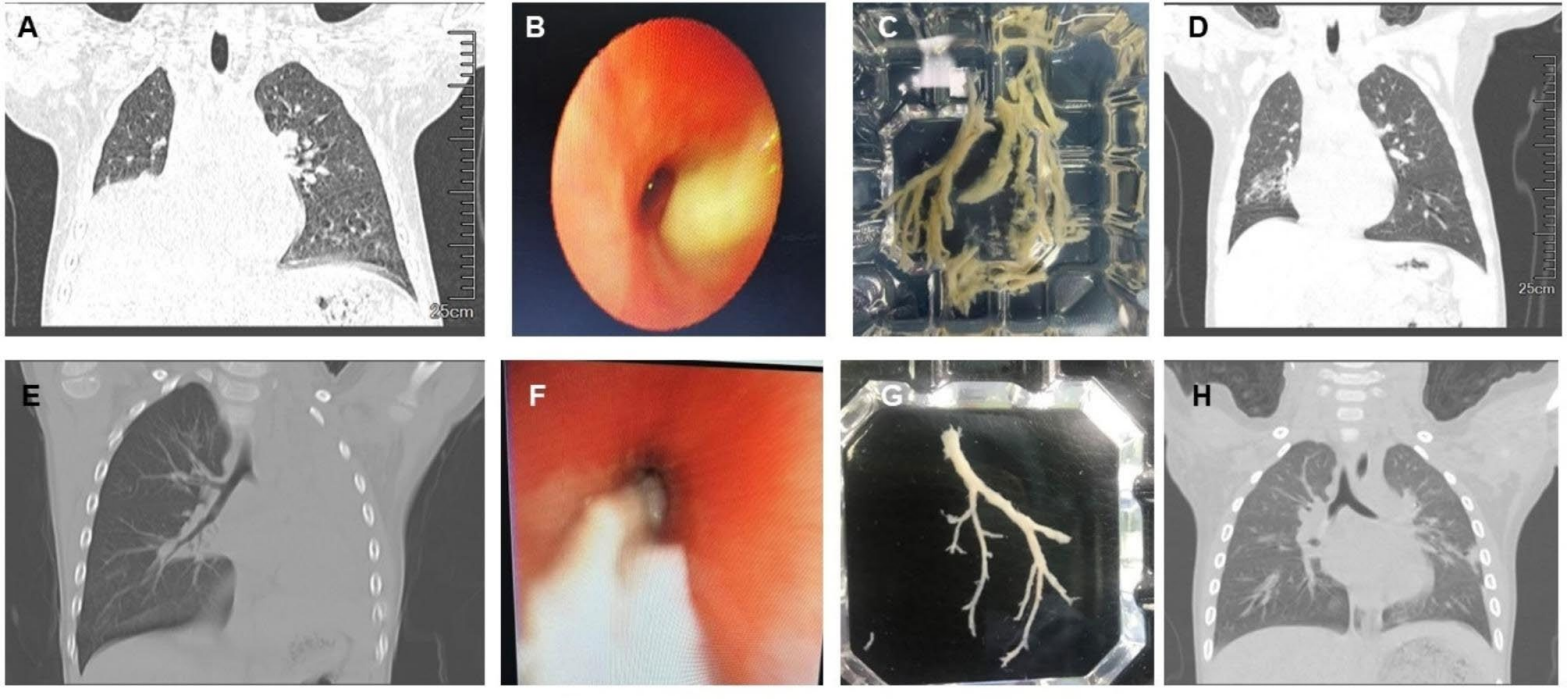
The relevant radiological and bronchoscopy images. Images A-D represent a 5-year-4-month-old girl diagnosed with MP Infection. A: Chest CT on admission showed large consolidated inflammation in the right lung, accompanied by atelectasis of the right lower lobe; B: Bronchoscopy revealed mucus plug obstructing the right lower lobe bronchus; C: The mucus plug was successfully removed; D: One month later, a follow-up chest CT showed almost complete resolution of the lung inflammation and complete lung re-expansion. Images E-H represent a 3-year-5-month-old boy diagnosed with Boca virus infection. E: Chest CT on admission showed high-density shadows and atelectasis in the left lung; F: Bronchoscopy revealed mucus plug obstructing the left main bronchus; G: The mucus plug was successfully removed; H: Eight days later, a follow-up chest CT showed complete lung re-expansion and almost complete resolution of the inflammation in the left lung
